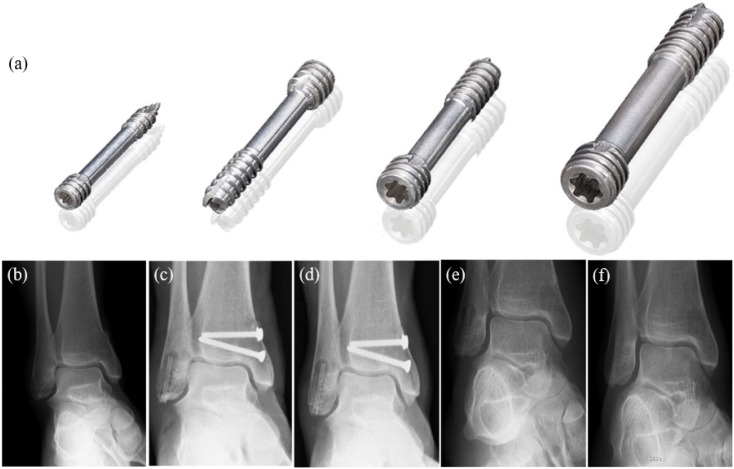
(a) MAGNEZIX® compression screws: from left to right: MAGNEZIX® CS 2.0, MAGNEZIX® CS 2.7, MAGNEZIX® CS 3.2, MAGNEZIX® CS 4.8. (b–f) X-ray of ankle fracture: (b) ankle fracture type AO-ASIF 44-A2.3; before surgery (c) six weeks after surgery; (d) 3 months after surgery; (e) 8 months after surgery, metal removal; (f) 17 months after surgery.^81^
